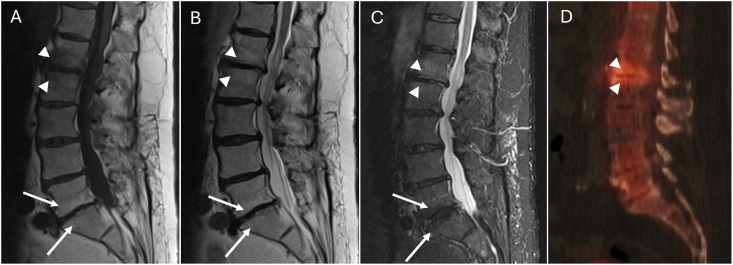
Example of partial discordance between MRI and SPECT-CT. Sagittal T1-weighted (A), sagittal T2-weighted (B) and STIR sequence (C) images demonstrate Modic type 1 changes at L1-L2 (arrowheads) and Modic type 2 changes at L5-S1 (arrows). At L5-S1, Modic type 2 changes are indicated by hyperintense signal in the vertebral bodies adjacent to the L5-S1 disc space on the T1-and T2-weighted images, with hypointense signal on the STIR image consistent with fatty replacement, and there is no significant associated radiotracer uptake. At L1-2, Modic type 1 changes are indicated by hypointense signal in the vertebral bodies adjacent to the L1-L2 disc space on the T1-weighted image, and hyperintense signal on the T2-weighted image consistent with marrow edema compared to the background signal in the rest of the vertebrae. At L1-L2 there is associated increased radiotracer uptake on sagittal reformatted bone SPECT-CT fused image (D).
